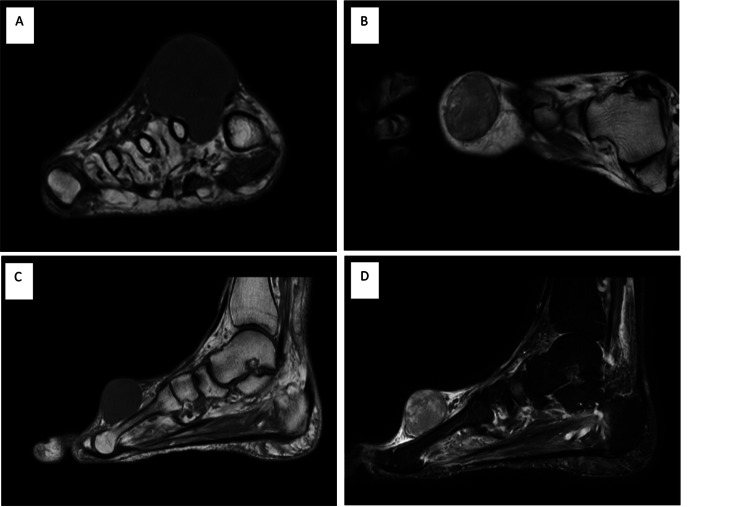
MRI of the dorsum soft tissue tumor. Coronal T1-weighted (A), transverse T1-weighted (B), and sagittal T1-weighted (C) images demonstrate the hypointense encapsulated mass. The sagittal T2-weighted image (D) demonstrates mixed intensity. MRI, magnetic resonance imaging
